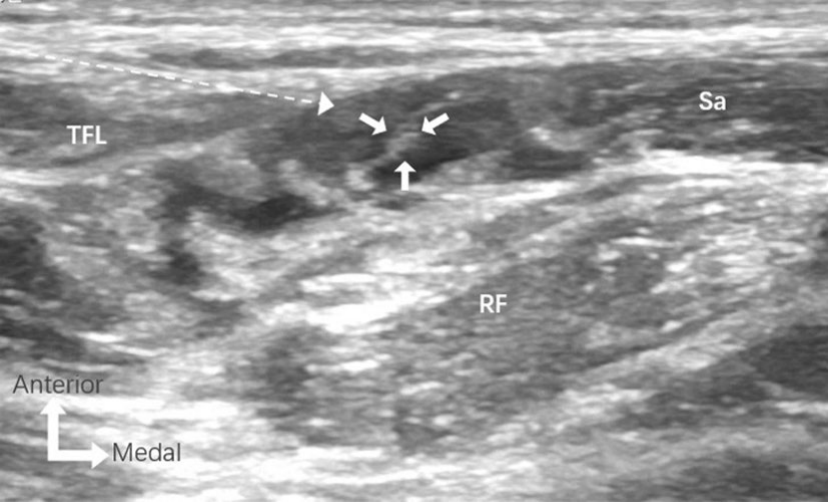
Images of ultrasound-guided lateral femoral cutaneous nerve (LFCN) block. The needle tip was positioned in the tunnel formed by the fascia between the sartorius and the tensor fascia lata. Dashed arrow, needle pathway; large arrows, LFCN; TFL, tensor fascia lata; RF rectus femoris; Sa, sartorius
